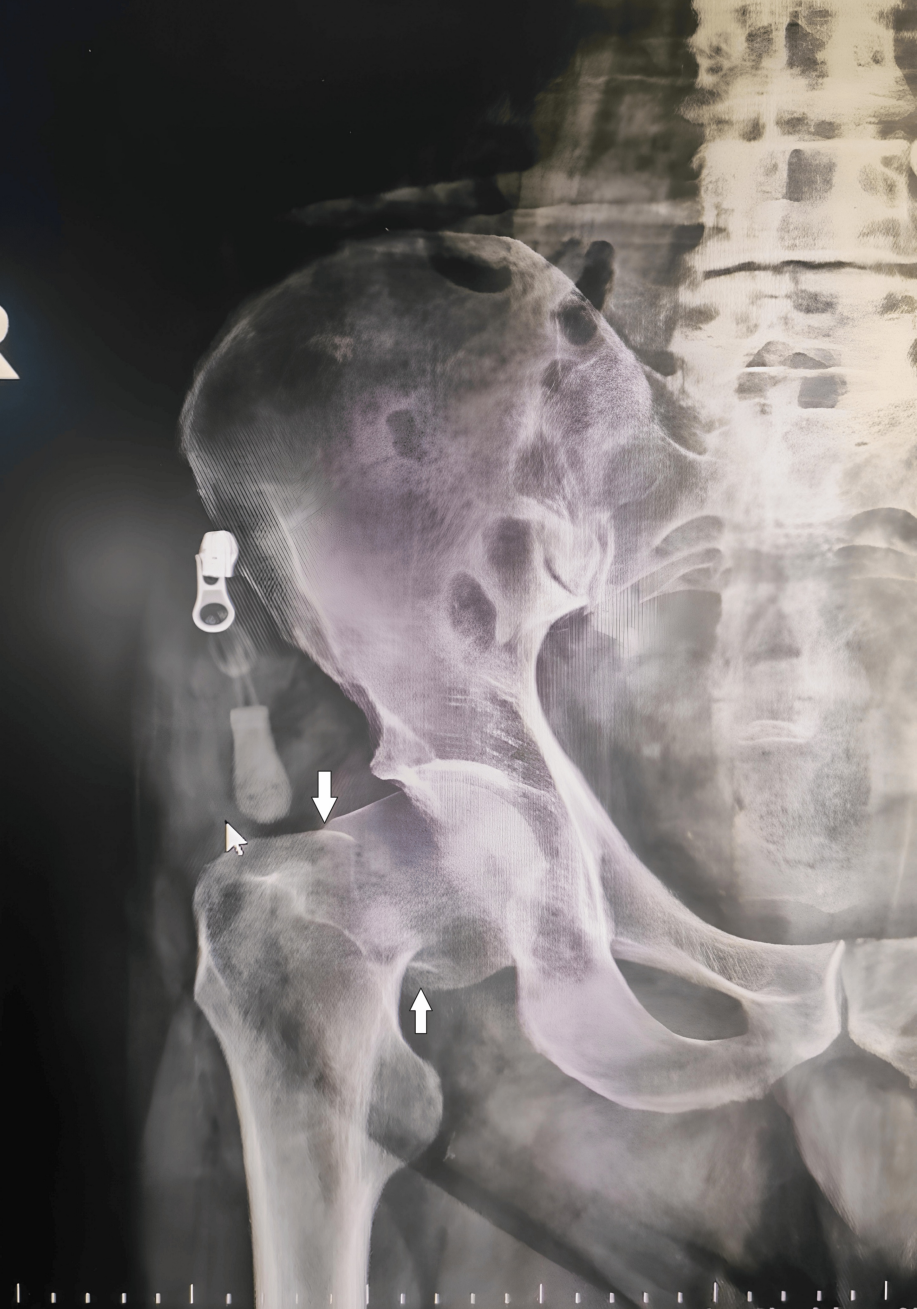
Anteroposterior pelvic radiograph showing a displaced right intertrochanteric fracture
